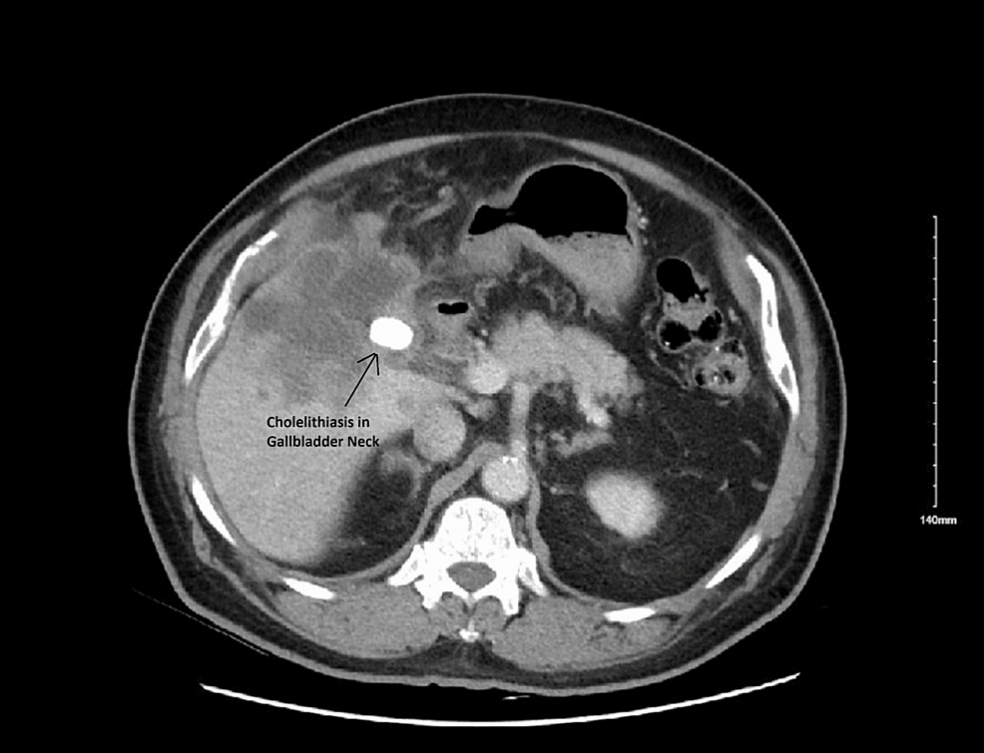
CT abdomen revealing cholelithiasis in the gallbladder neck along with hepatic mass.
